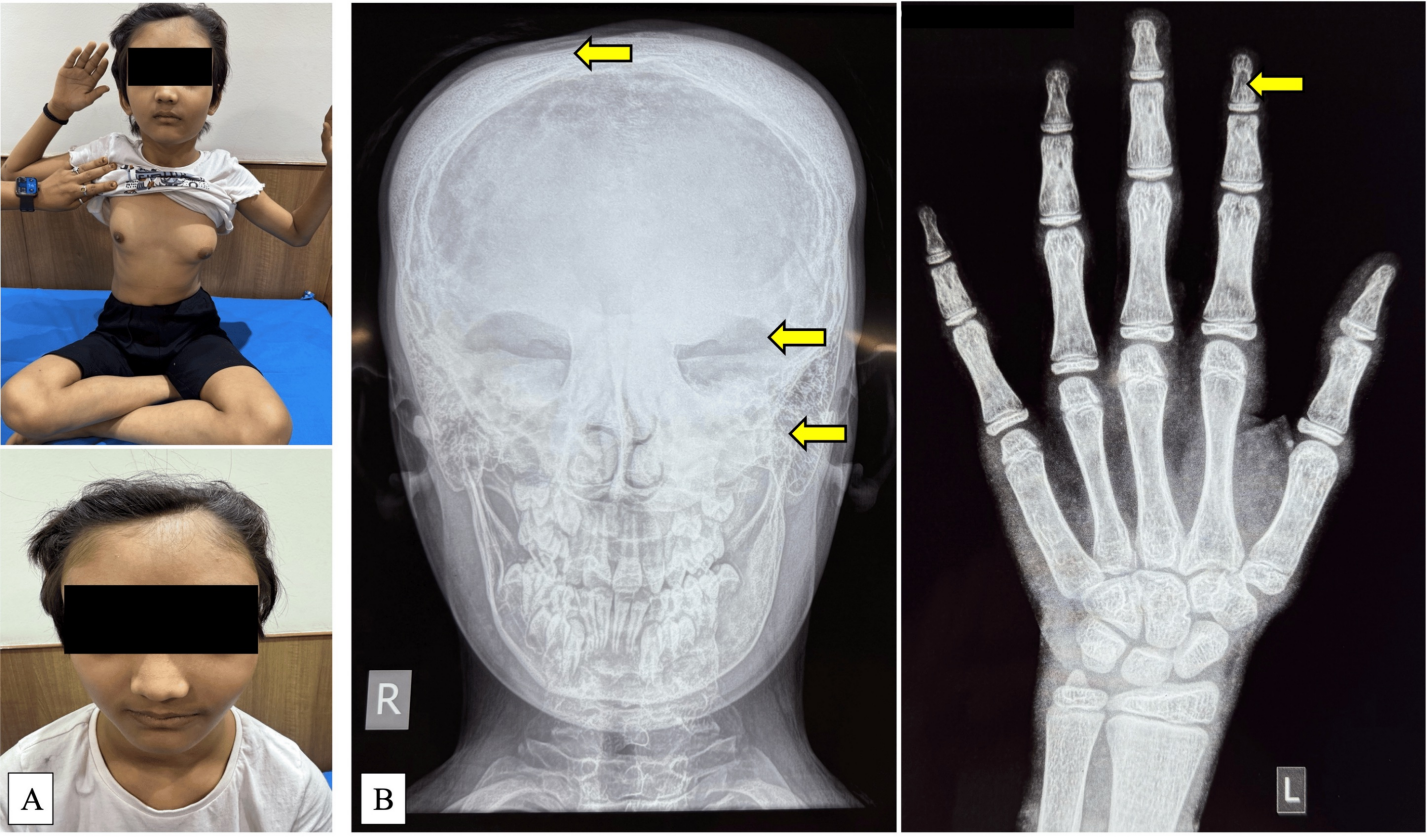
8.3-year-old girl with McCune-Albright syndrome. A. Bilateral breast development, stage 3/4, and facial asymmetry (craniofacial fibrous dysplasia). B. Skull radiograph showing craniofacial fibrous dysplasia and multiple cystic lesions on left-hand bone age radiograph.
